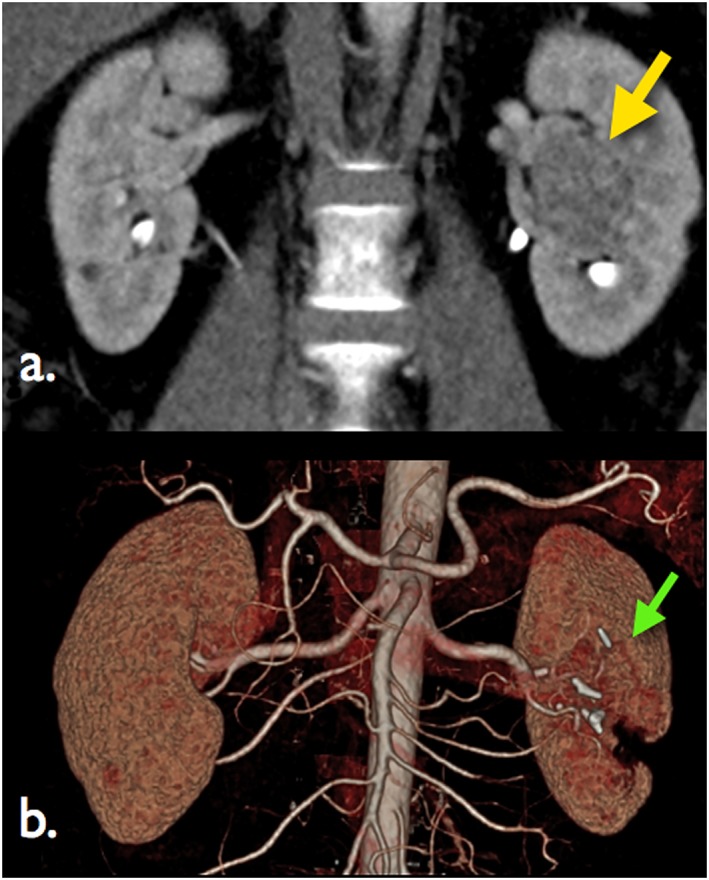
(a) Saggital section of complex hilar tumour (yellow arrow). (b) Post‐operative 3D reconstruction computed tomography scan to show scar defect in kidney (green arrow) post‐RALPN
